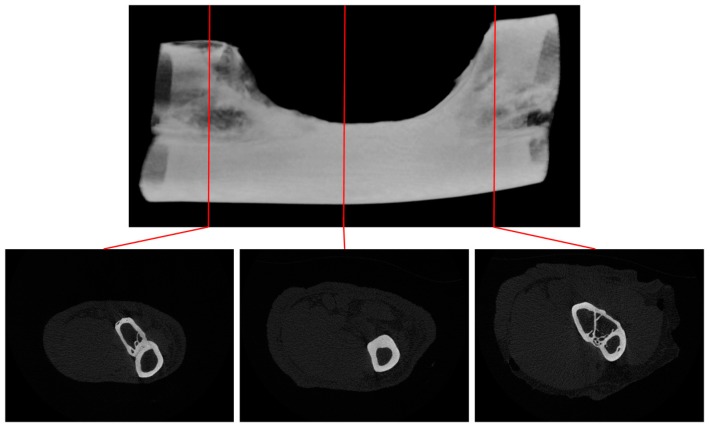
20 mm defect after 1 year of healing time micro CT-scanning. There is no restoration in most of the defect volume. Deformation in the cortex is revealed in the segmental end of ulna, where there is a fusion of the radius and ulna. Inside the defect there is a change in anatomy of the radius, resulting in a thicker cortex.
